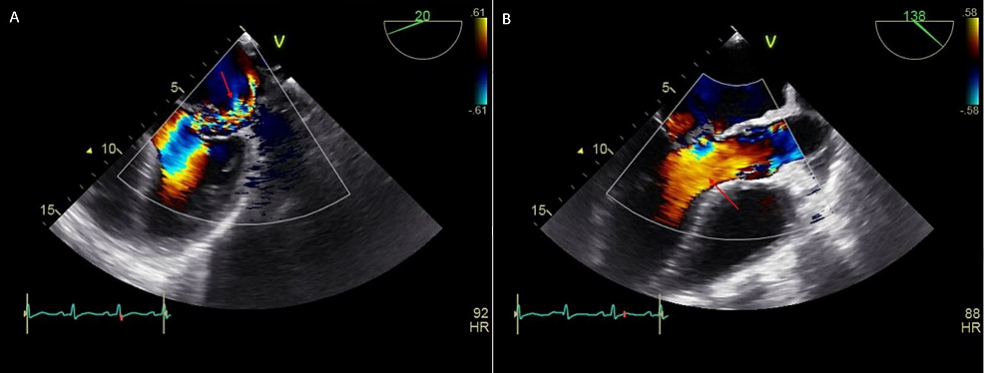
Color Doppler Transesophageal echocardiogram (TEE) demonstrating moderate-severe mitral regurgitation (A) and moderate aortic regurgitation (B)
